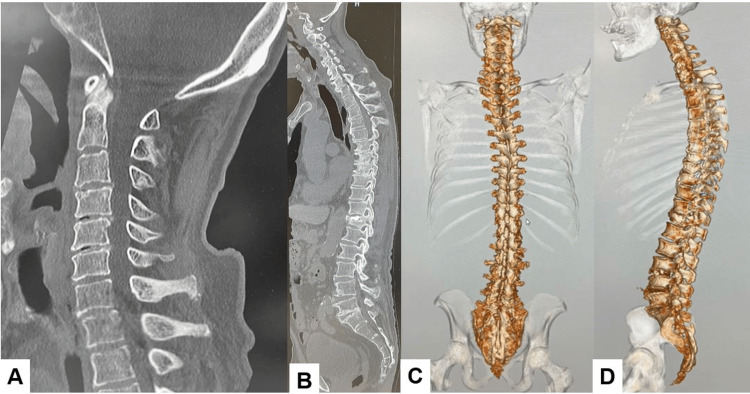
Preoperative CT. A. Cervical mid-sagittal reconstruction CT. B. Whole spine posterior 3D CT. D. Whole spine lateral 3D CT.
